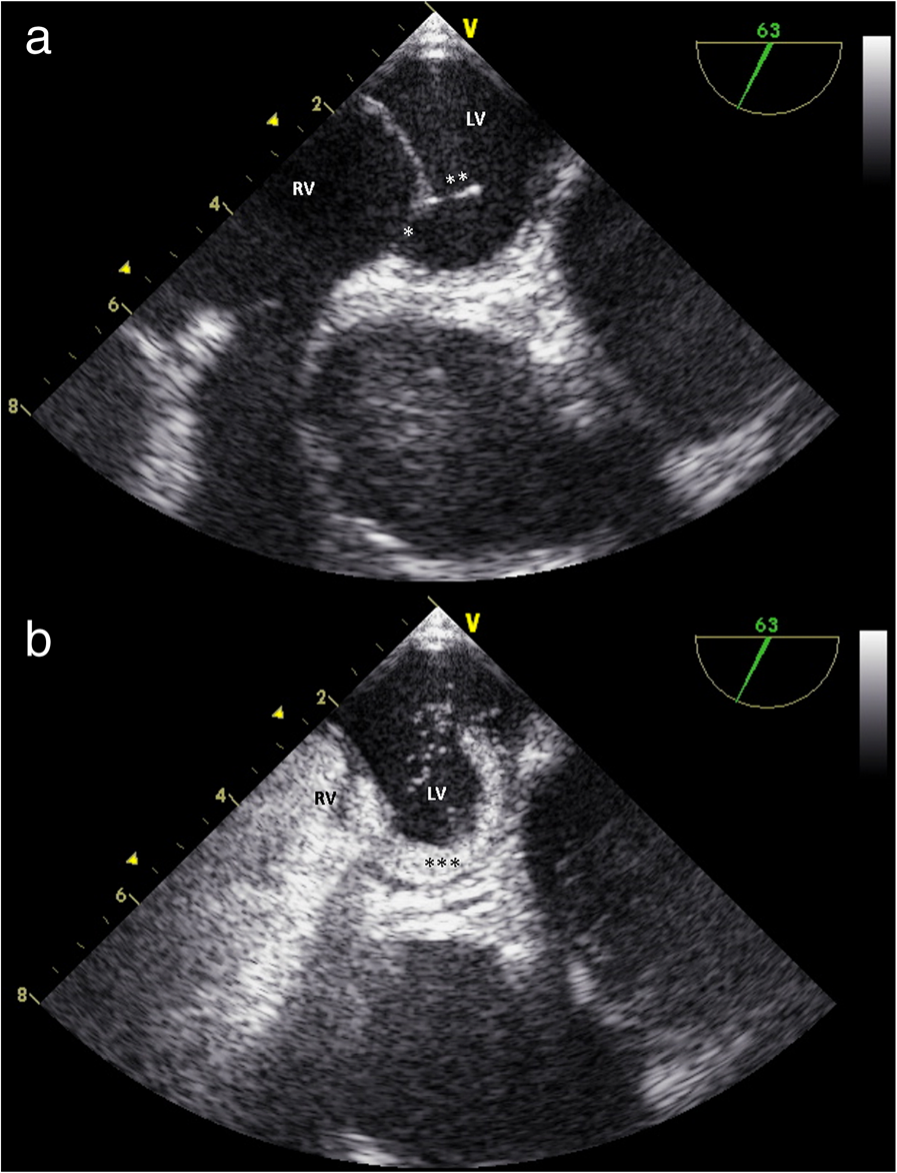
a and b: Transoesophageal echocardiography revealed a PFO. LV left ventricle; RV right ventricle; * PFO, 8 mm;  pacemaker lead; * contrast agent.
